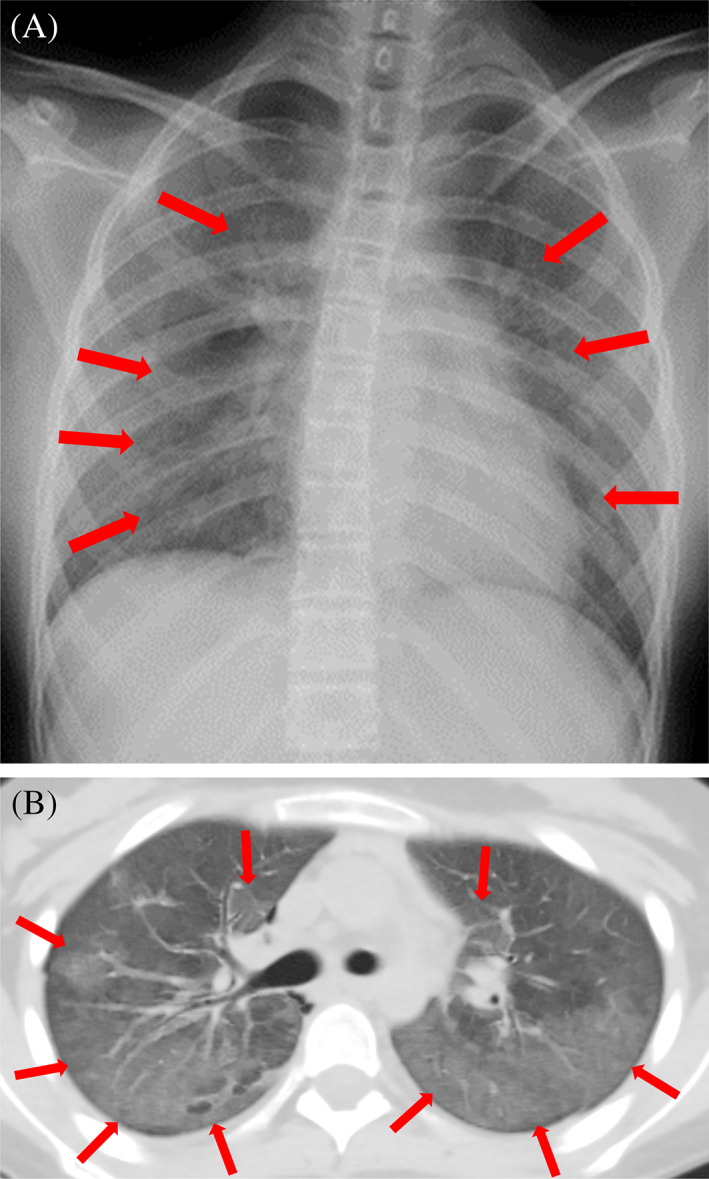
Imaging findings for a patient with *Pneumocystis jirovecii* pneumonia (PJP) after CD19‐targeted chimeric antigen receptor T‐cell therapy. (A) Chest radiography shows diffuse ground‐glass opacities indicated by red arrow marks in both lungs. (B) Chest computed tomography also shows diffuse ground‐glass opacities indicated by red arrow marks in both lungs, especially the dorsal regions. These findings are typical radiological features of PJP.
